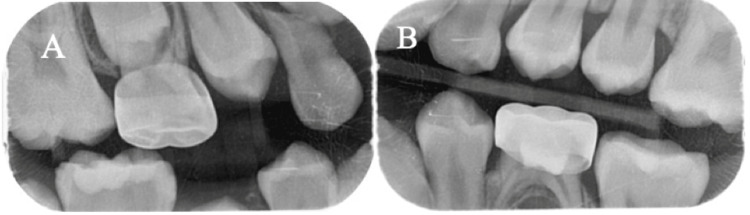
(A, B) Six-month postoperative radiographs showing proper alignment and eruption pathways of the permanent teeth, with no residual signs of the odontoma or retained primary teeth, and radiographic confirmation of healthy bone remodeling and integration around the surgical site.
